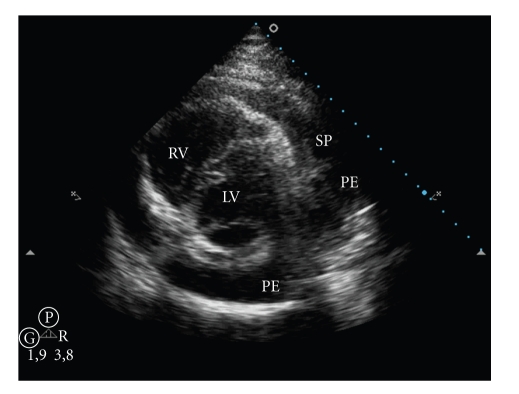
Transthoracic echocardiography: short axis view showing large pericardial effusion with septations (PE: Pericardial effusion; SP: septations; LV: left ventricle; RV: right ventricle).
